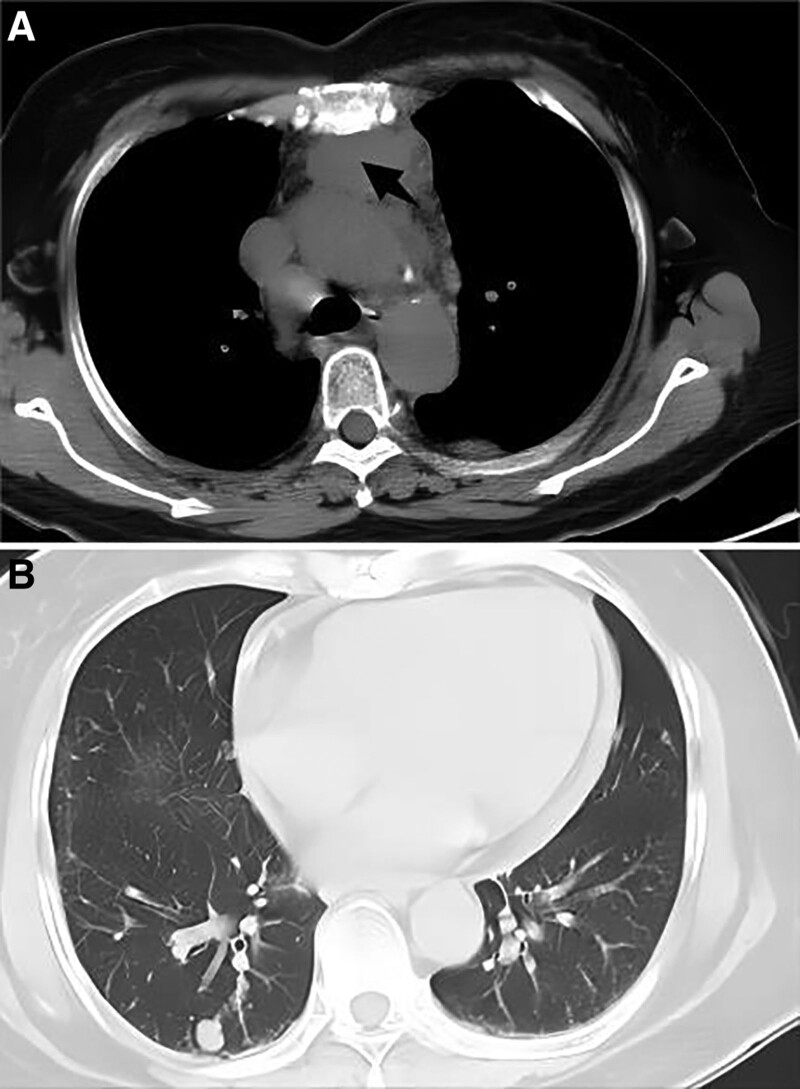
High-resolution CT image of chest. (A) Anterior mediastinal mass, considering thymoma (black arrow). (B) Multiple nodules in bilateral lung and multiple lymph nodes in mediastinum. CT = computed tomography.
